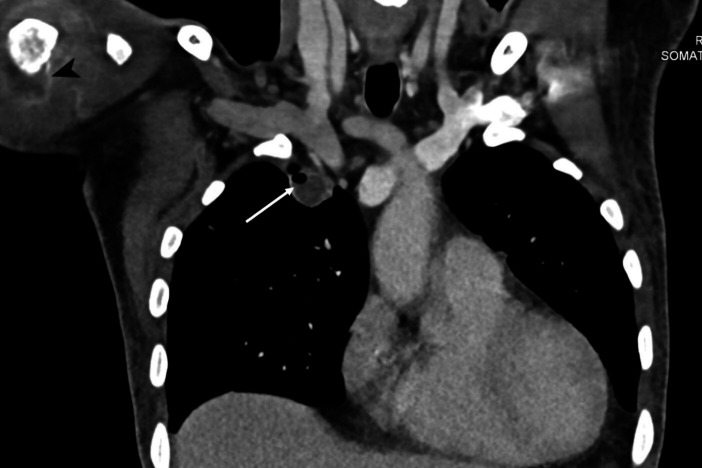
Computed tomography image (coronal view) showing a small lung abscess with air–fluid level (arrow) in the upper lobe of the right lung. Also, note the periarticular abscess around the right shoulder joint (arrowhead) that resulted from septic arthritis.
